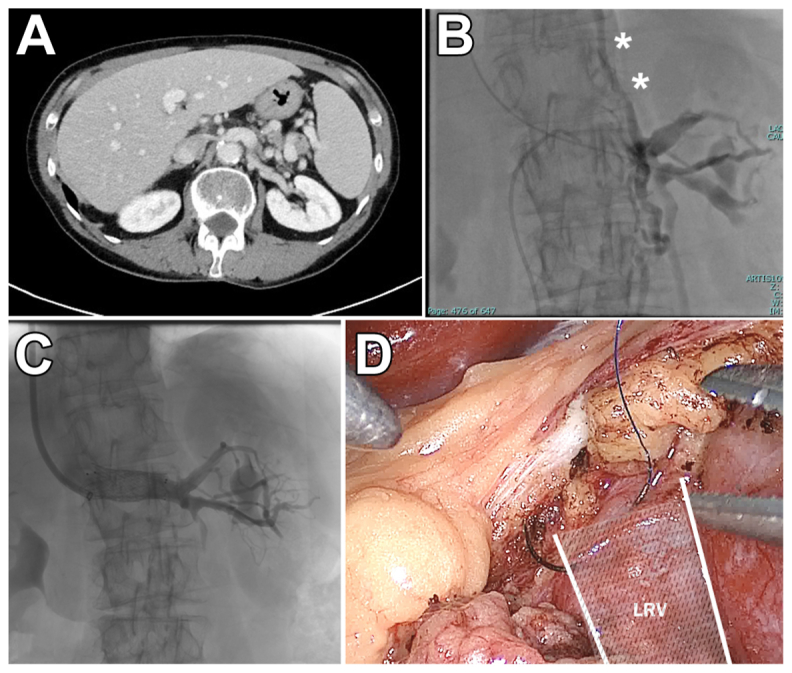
A preoperative computed tomography scan revealed a recurrent Nutcracker syndrome onset (*A*).
